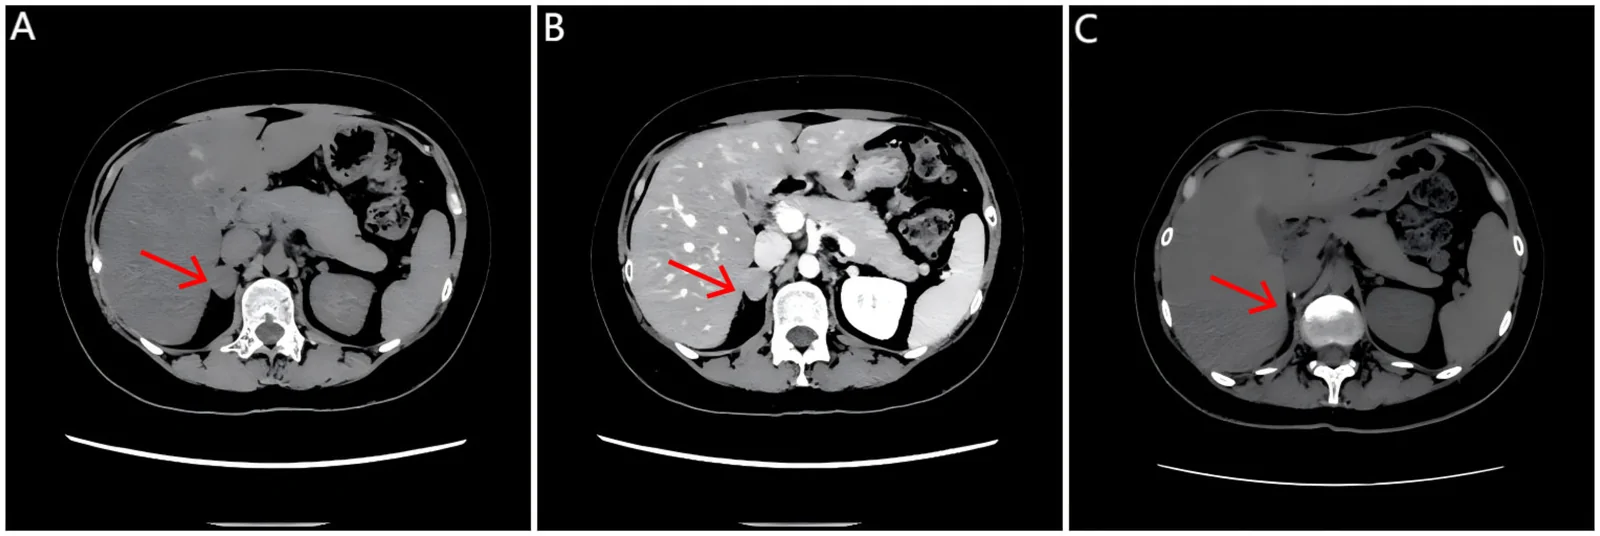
Adrenal CT imaging. (A) Preoperative plain CT scan showing a 20 mm × 18 mm nodule in the right adrenal gland (arrow). (B) Enhanced CT scan demonstrating significant enhancement of the right adrenal nodule (arrow), indicative of adrenal adenoma. (C) Postoperative plain CT scan (7 months after surgery) showing changes in the right adrenal gland consistent with surgical removal of the adenoma (arrow).
